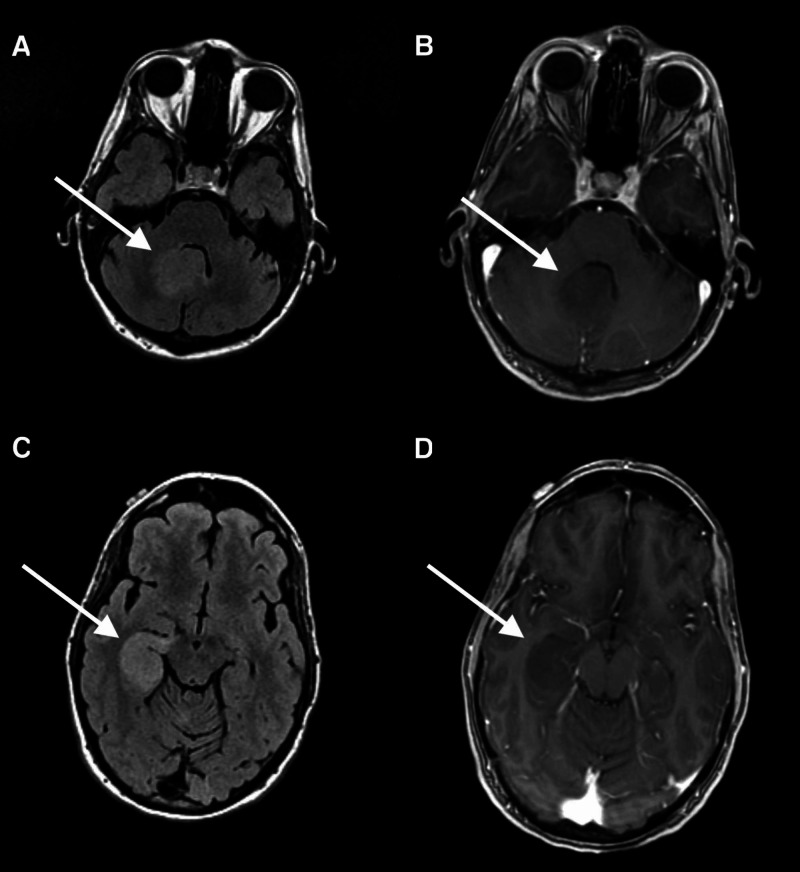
Axial FLAIR and T1 MRI with contrast demonstrating non-enhancing FLAIR hyperintense masses in the right cerebellum (Image A, B) and mesial right temporal lobe (Image C, D) compatible with multifocal Grade III astrocytoma FLAIR: fluid attenuated inversion recovery
